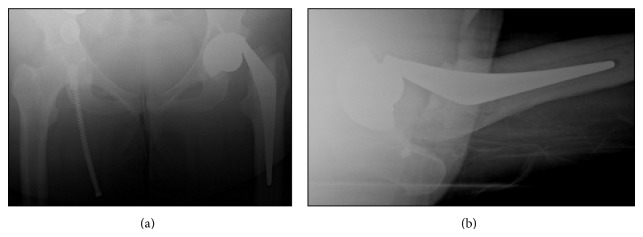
(a) AP and (b) lateral radiographs of the dissociated THA.
